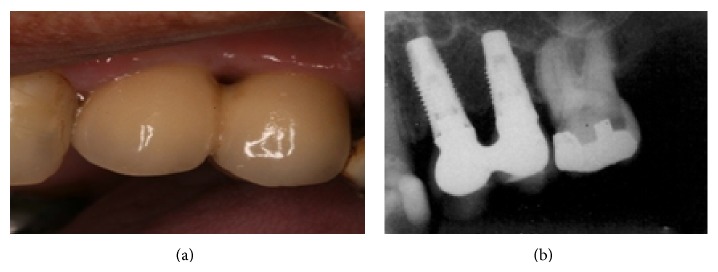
Six-year follow-up: clinical view of the implant-supported fixed prosthesis (a) and periapical radiograph of implants showing no bone loss (b) (May 2014).
